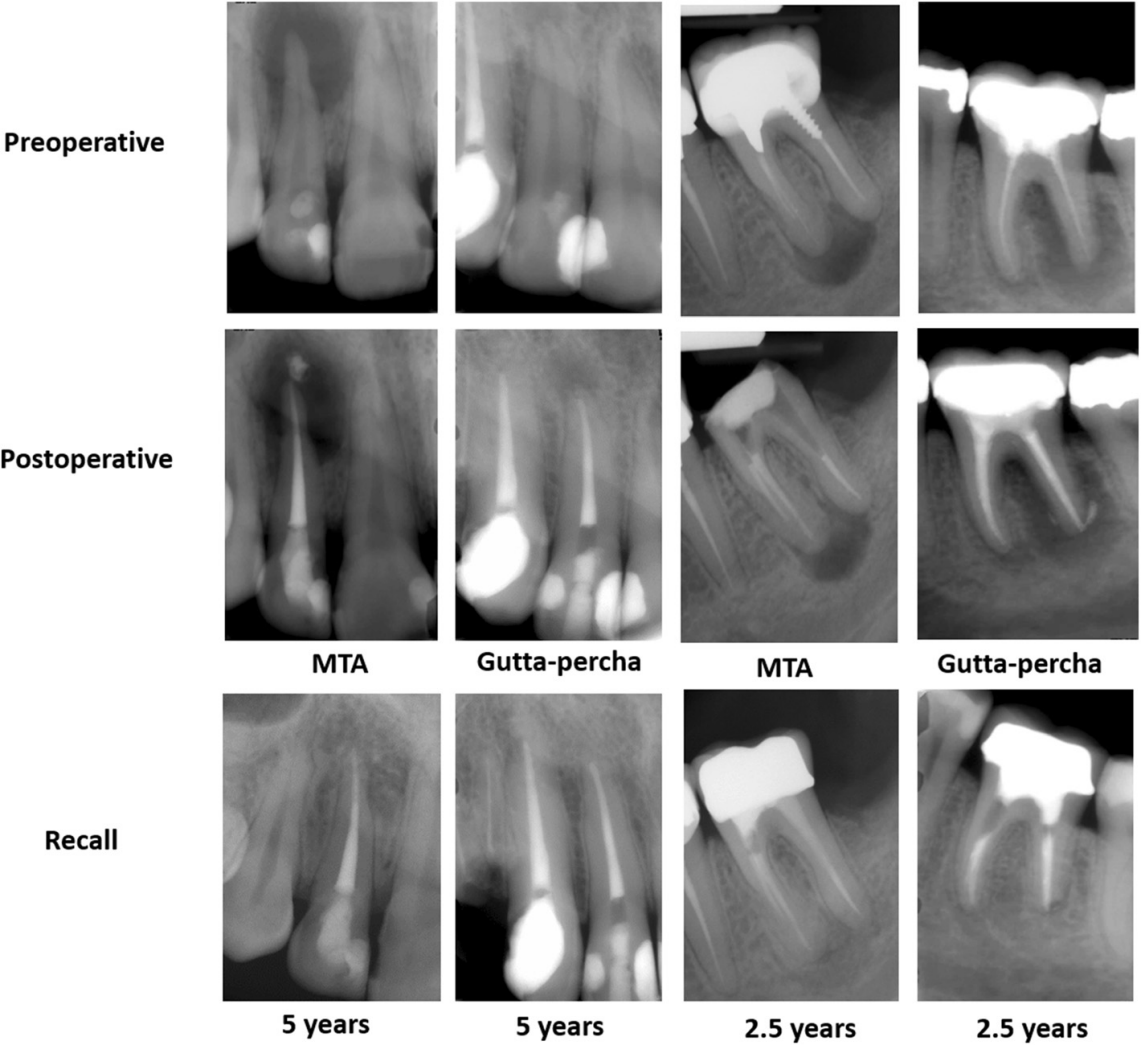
Periapical radiographs of primary treatment in maxillary lateral incisors and secondary treatment in mandibular first molars followed for 5- and 2.5-years
